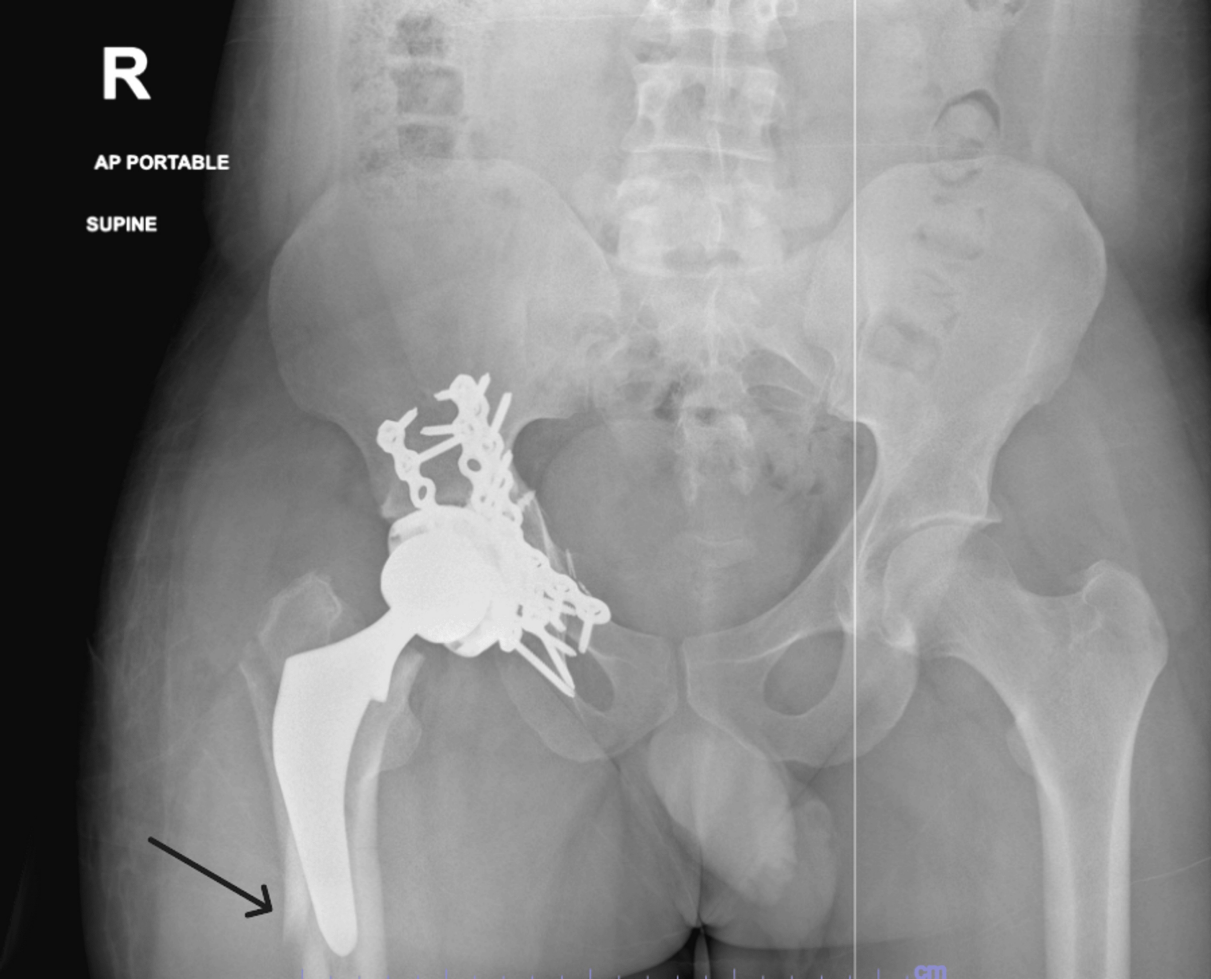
Preoperative AP X-ray of the pelvis demonstrating periprosthetic fracture of the femoral shaft Arrow indicated fracture around the prosthesis. AP, anteroposterior
